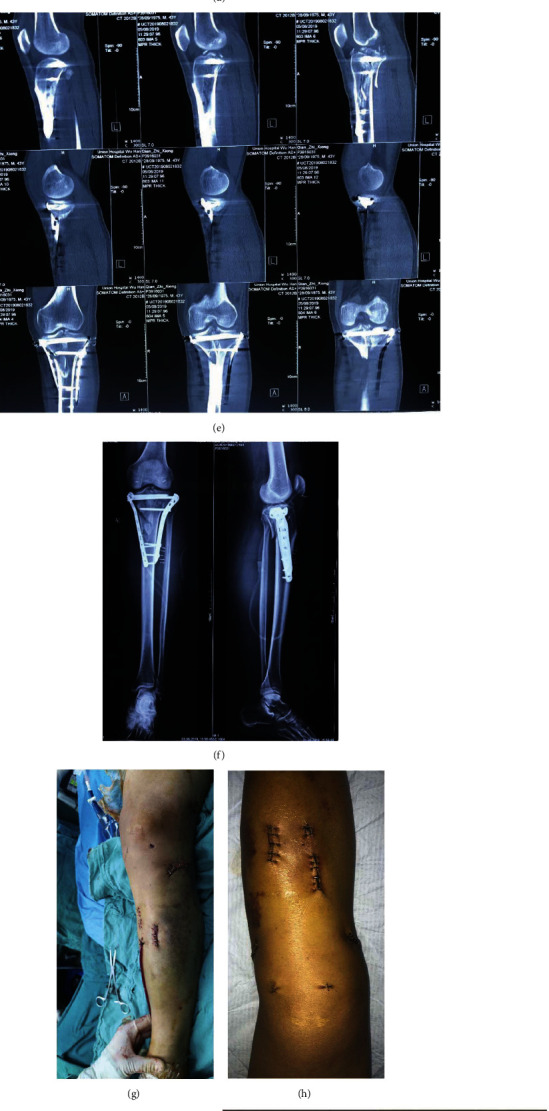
A 43-year-old male with Schatzker type VI tibial plateau fracture with spur sign. (a, b) X-rays of tibial plateau fracture presurgery. (c–e) Preoperative CT imaging results of the tibial plateau fracture. (f) X-rays of tibial plateau obtained after operation. (g, h) Intraoperative and 3 days postoperative incisions of the entire injured limb.(i) Application of double-reverse traction repositor (DRTR) during operation. (j–m) Intraoperative fluoroscopic X-ray images of the fracture sites before and after the application of the traction device.
